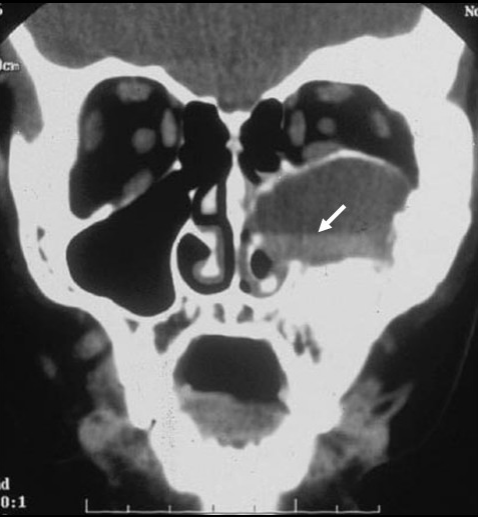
Fluid-filled cyst in FD. True fluid-filled cysts can occur in FD lesions. Shown is the CT scan of fluid-filled cyst that arose in a 12 year old boy with MAS who presented with facial nerve parasthesias and displacement of the orbit that occurred over approximately one week. The fluid-filled nature of the lesion is demonstrated by the fluid-fluid level (arrow). These lesions are more frequent in the craniofacial bones and can be aggressive. They usually require prompt surgical intervention.
